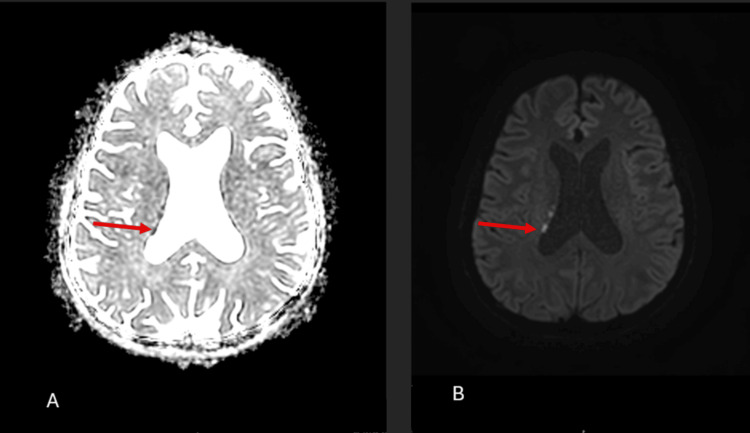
MRI of the head (A) Axial T1-weighted view. (B) Diffusion-weighted imaging view. Multiple bilateral deep cerebral parenchymal areas of restricted diffusion are noted, involving the posteromedial temporal lobes, both thalami, the posterior limbs of the internal capsules, and the deep parietal juxtaventricular regions (red arrows).
